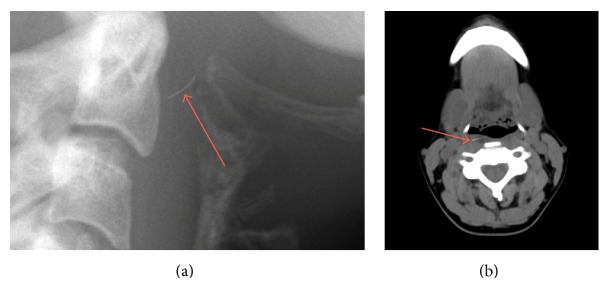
(a) Lateral neck film showing a thin linear density projecting over the base of the dens, with associated prevertebral soft tissue swelling. (b) Nonenhanced axial neck CT with slice thickness of 2 mm demonstrating a 12.3 mm × 1.0 mm linear hyperdense structure in the right posterior pharyngeal wall.
